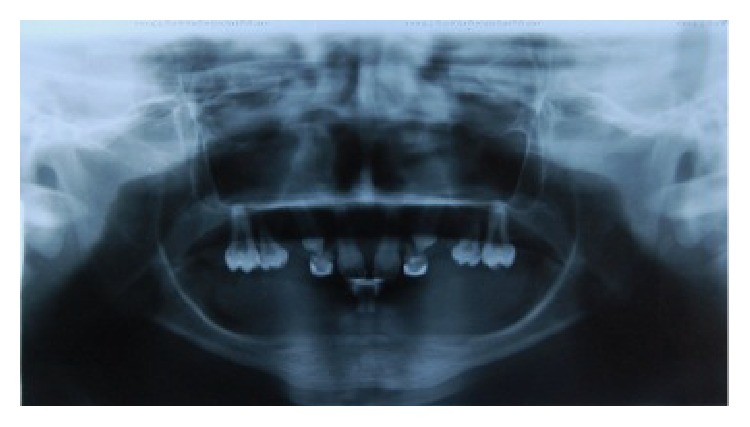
Panoramic radiograph view: prolonged retention of elements 52, 55, 62, and 65. The root resorption of teeth 52 and 62 can be observed, as well as the impaction of elements 13 and 23.
